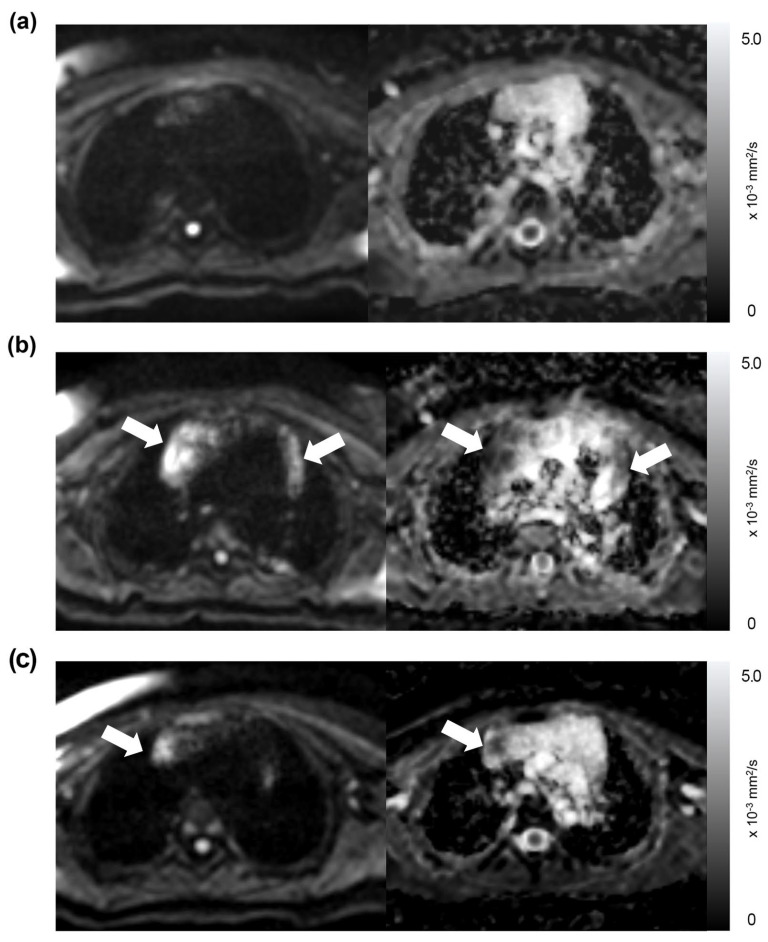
Changes in the presence and pattern of restricted diffusion observed in follow-up MRIs of a girl with a history of mediastinal neuroblastoma. (a) The MRI performed when the girl was 17 months old showed the thymus exhibiting unrestricted diffusion (Philips MRI, treatment status: on-treatment (chemotherapy), and thymus size: diminished) (b) The next MRI, performed 4 months later, revealed a multifocal pattern (arrows) of restricted diffusion (Siemens A MRI, treatment status: off-treatment (post-treatment), and thymus size: prominent). (c) Another follow-up MRI, performed 8 months after the second one, showed a focal pattern (arrow) of restricted diffusion (Philips MRI, treatment status: off-treatment (post-treatment), and thymus size: prominent).
